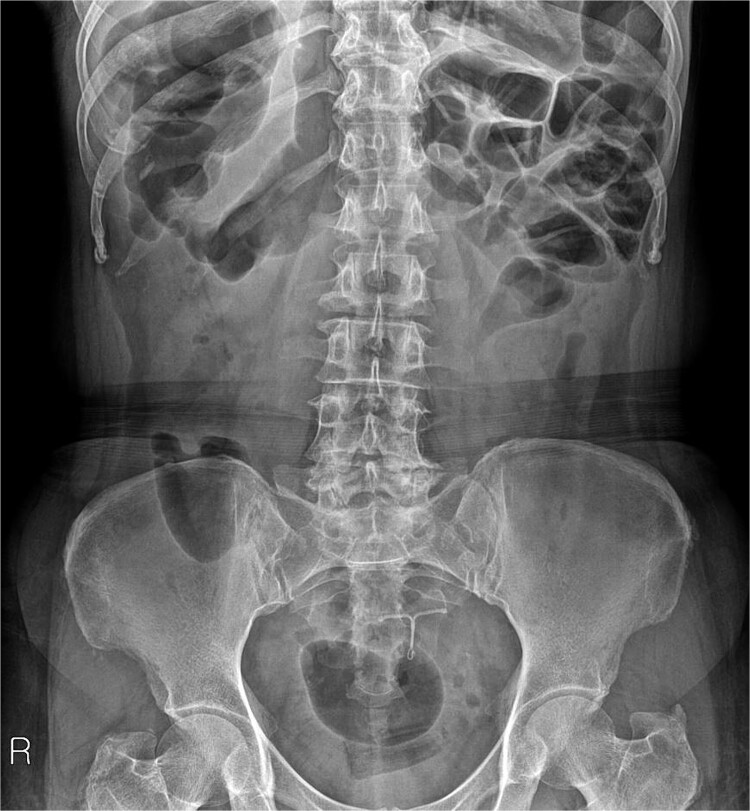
IUD identified on abdominal simple X-ray.
